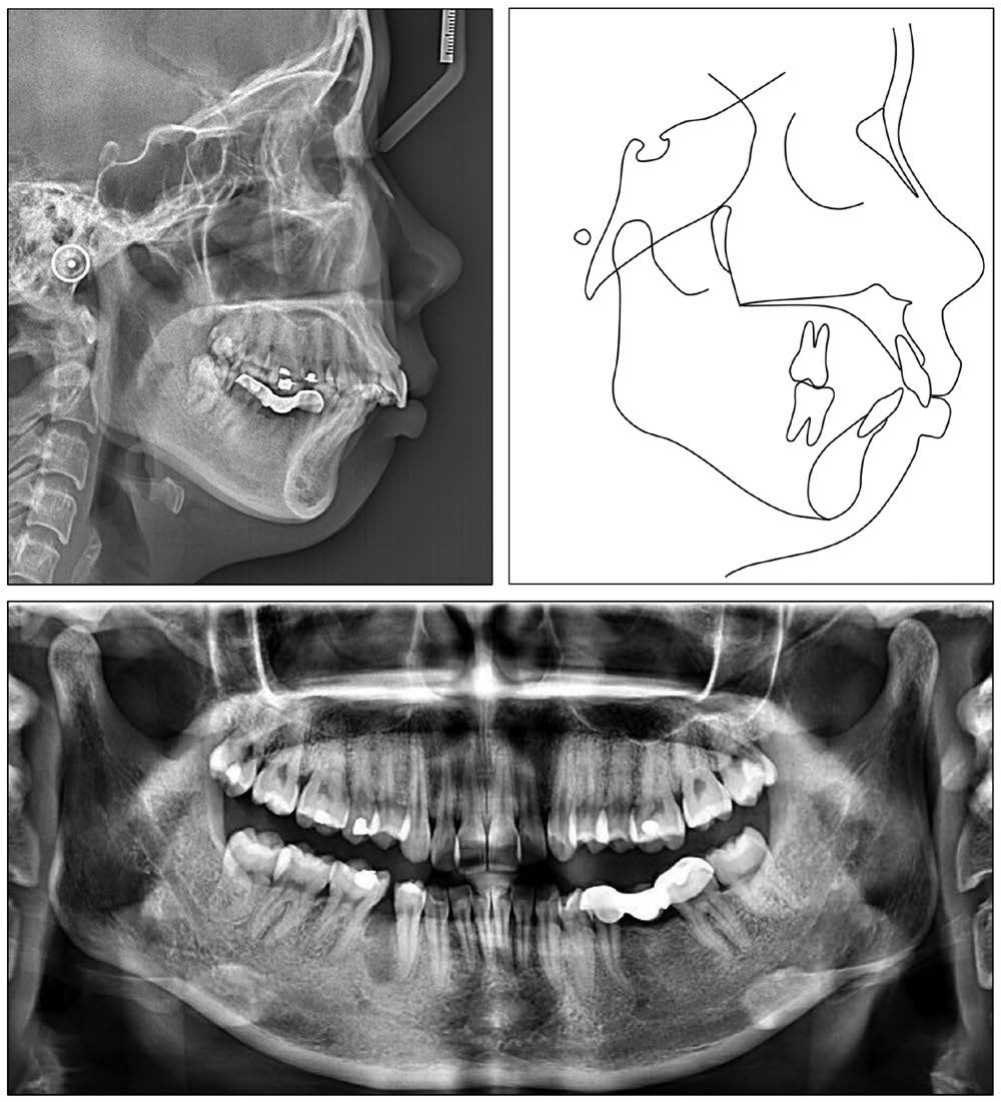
Pretreatment panoramic and cephalometric radiographs and tracing.
